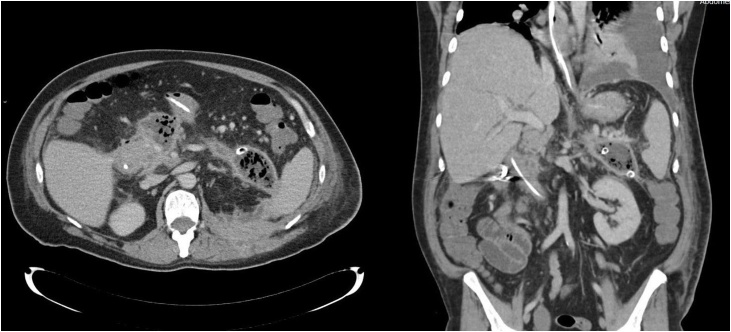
CT scan of the abdomen after drainage. This scan shows still the presence of a pancreatitis and ongoing necrotic tissue.
